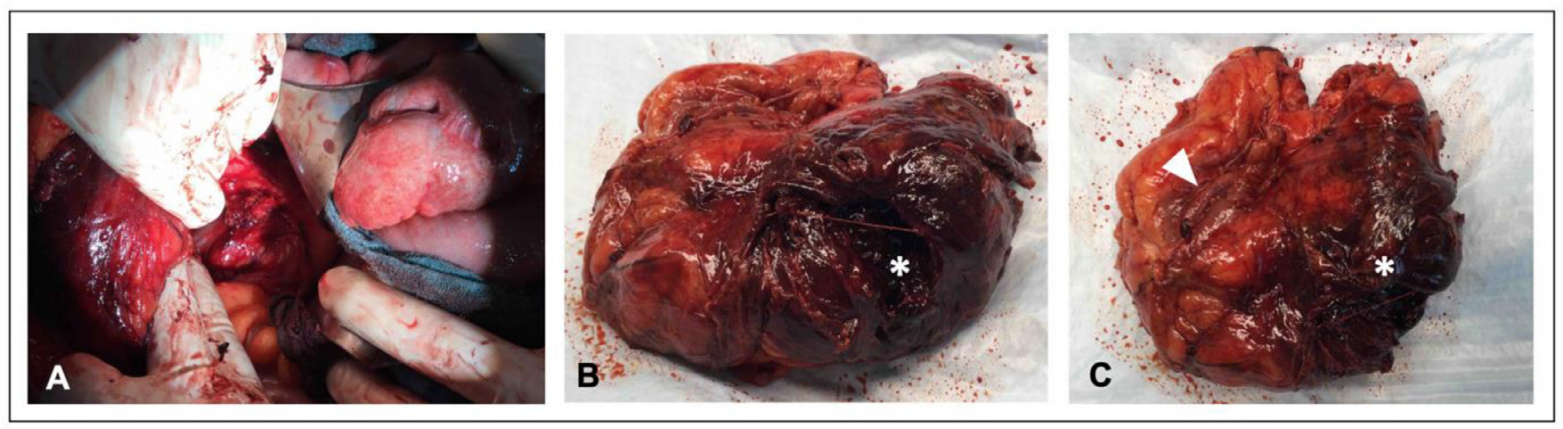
Images documenting the nephrectomy procedure and the removed kidney. (A): Intraoperative photograph showing the surgical site. (B, C): Photographs of the removed kidney. * marks the hematoma identified in the CT scan, and ► indicates part of the metal coils from the endovascular embolization.
